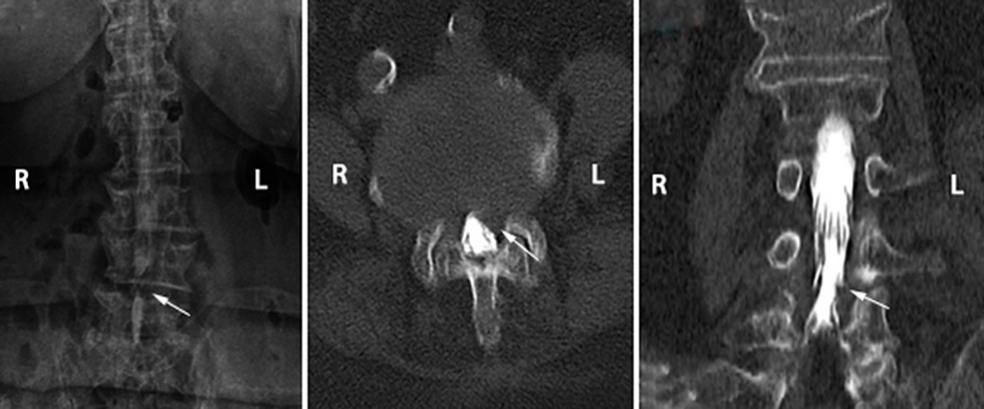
The patient who had a complaint of low back and left leg pain. Root compression at the left L4-L5 level was observed in myelography and CT myelogram. MRI could not be performed due to orthopedic plaque in the tibial bone.
